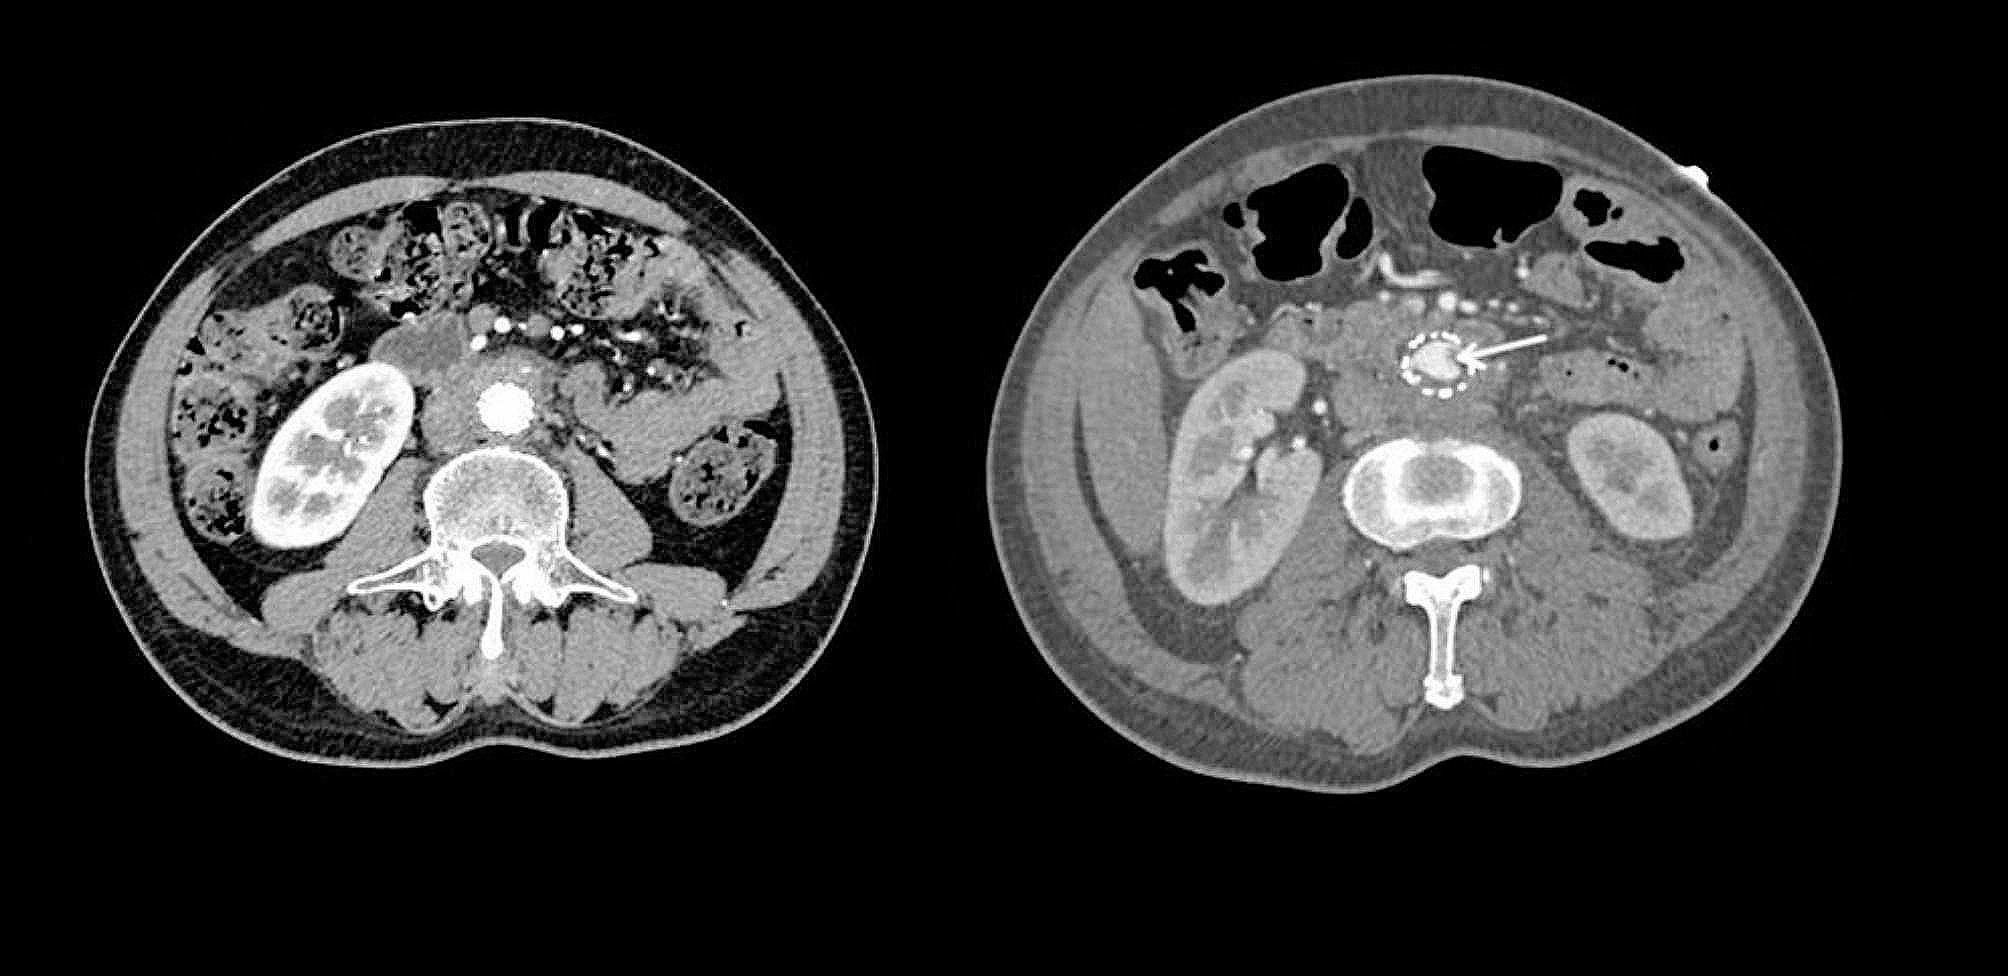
The left image shows the first rechecked CTA on August 27th, 2020. The right image shows intra-stent mural thrombosis and peri-stent soft tissue shadow
